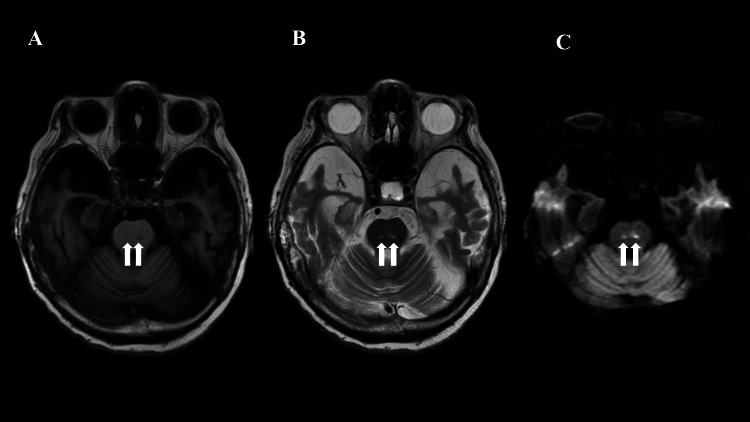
MRI findings at the time of hospitalization. Small abnormal lesions of the pons showed hypointensity in T1W (A) and hyperintensity in T2W (B) and DWI (C). T1W: T1-weighted image; T2W: T2-weighted image; DWI: diffusion-weighted image
